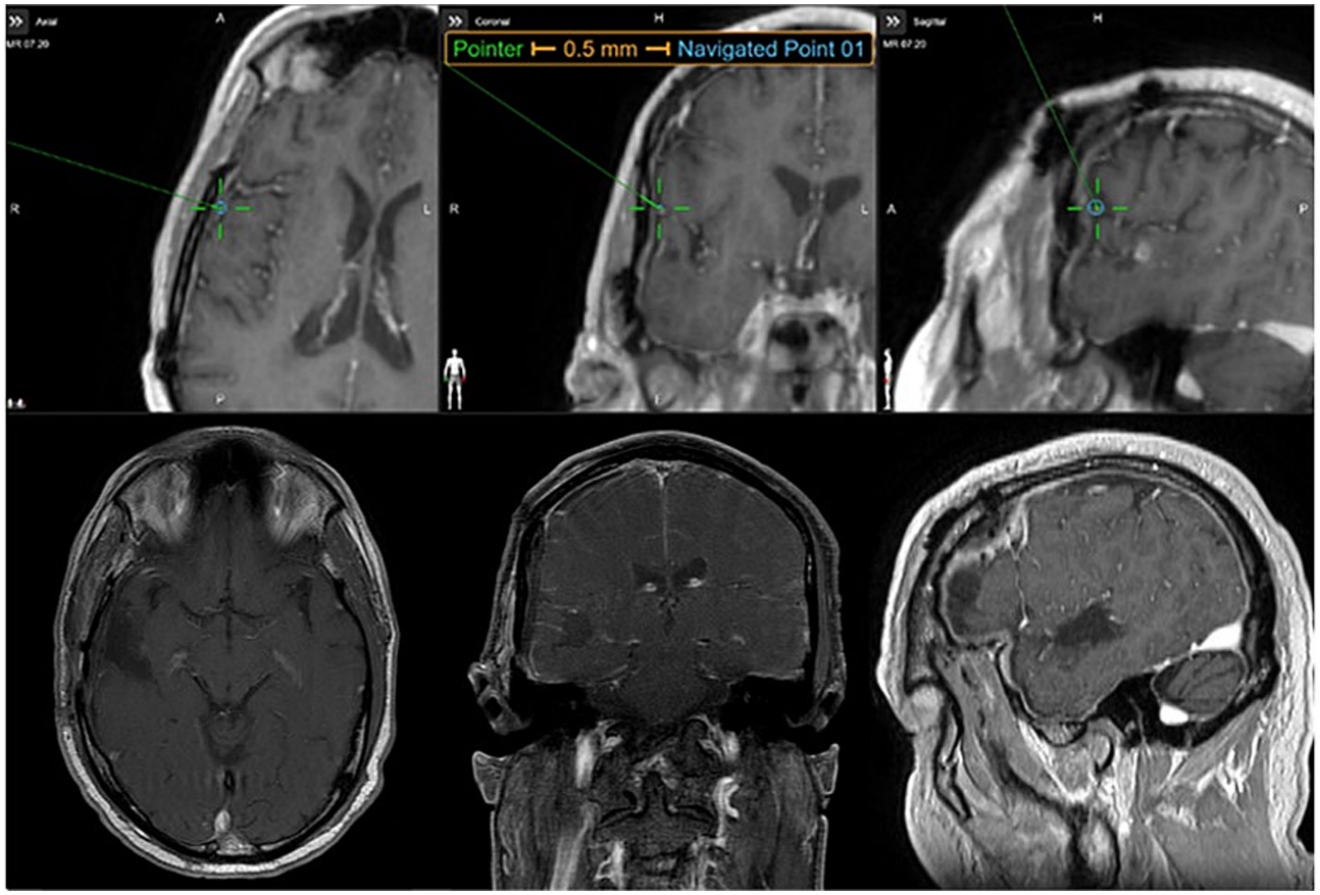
Intraoperative mapping with positive language findings in the right hemisphere and postoperative MRI. All scans are displayed in radiologic convention. (A) Positive language findings in the right frontal lobe when the patient exhibited speech arrest at 10 mA during direct electrical stimulation (DES) and (B) postoperative MRI showing a gross total resection of the recurrent glioblastoma.
